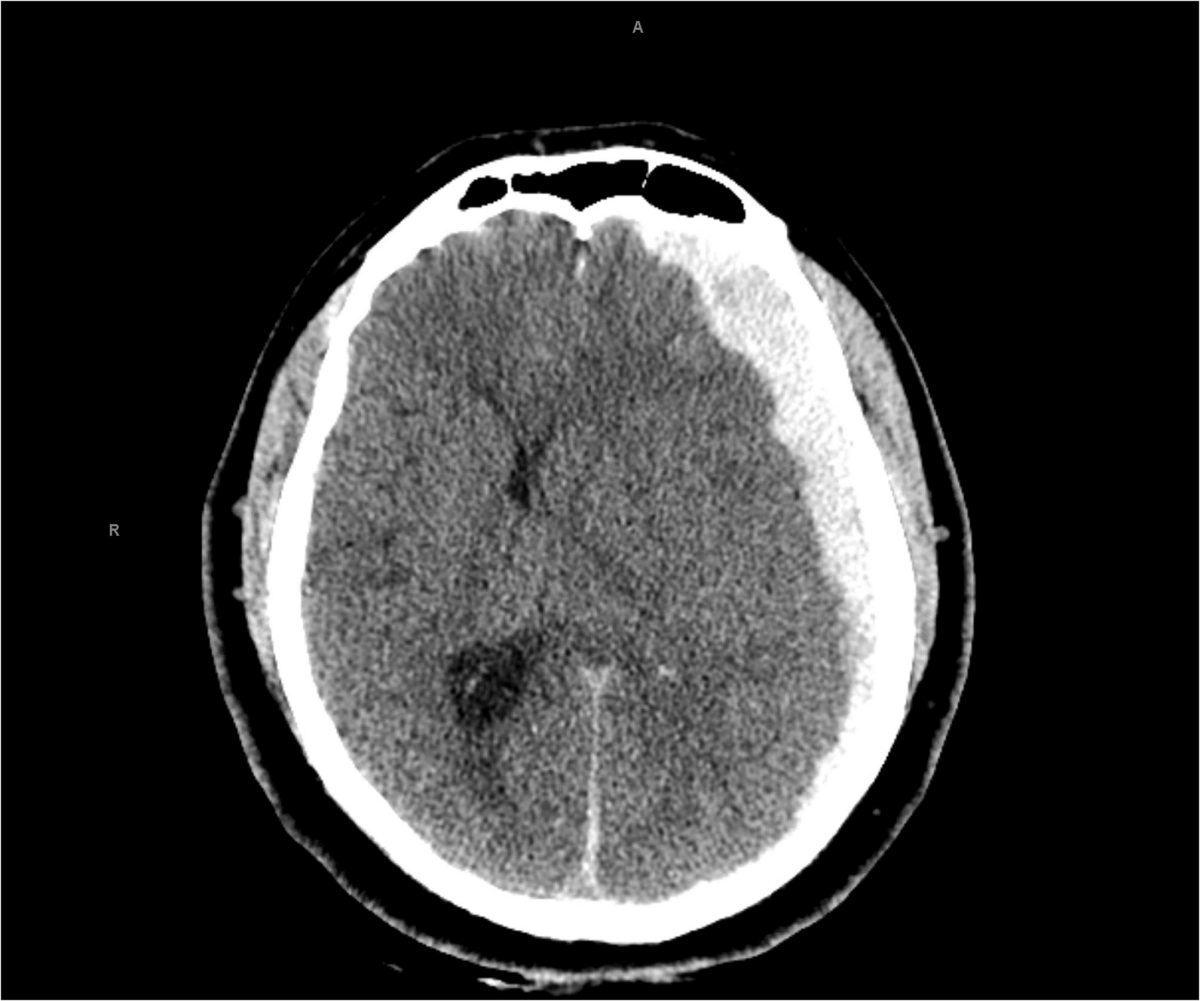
Computed tomography head showed an acute left subdural hemorrhage with significant left-to-right midline shift.
